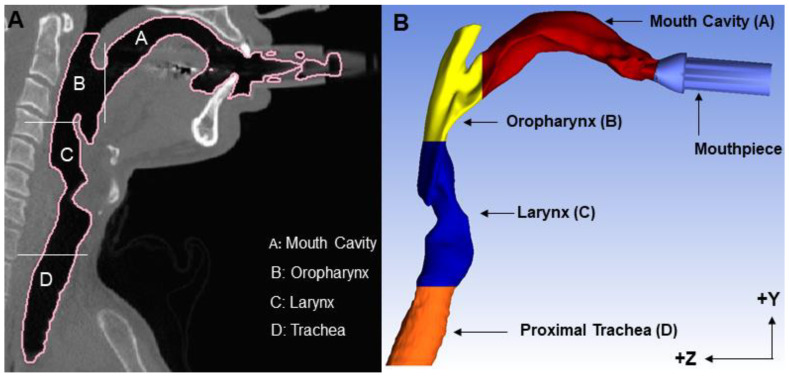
Definition of the subregions of the upper airway (A) CT scan of the oral airway of subject H1. (B) Reconstructed 3D model. The mouth is the oral cavity that extends from the back of the teeth to the uvula. The oropharynx region is the cavity from the back of the uvula to the tip of epiglottis. The larynx region is from the tip of the epiglottis to below the vocal cords and top of the trachea. The Z direction indicates the direction of gravity.
